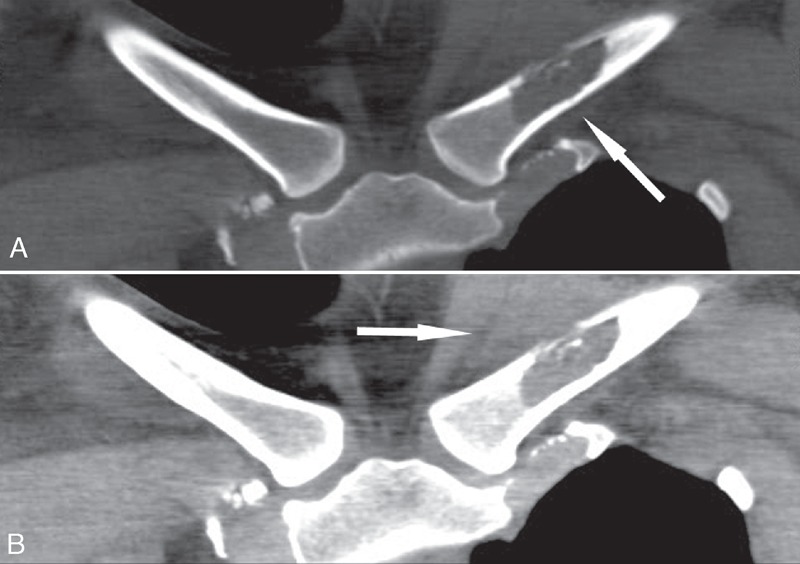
CT multiplanar reconstruction images show (A) an osteolytic lesion (arrow) with bone residual of the bone marrow and a disruption of the upper cortex in the diaphysis of the left clavicle and (B) surrounding tumor extension and soft tissue edema (arrow). CT = computed tomography.
